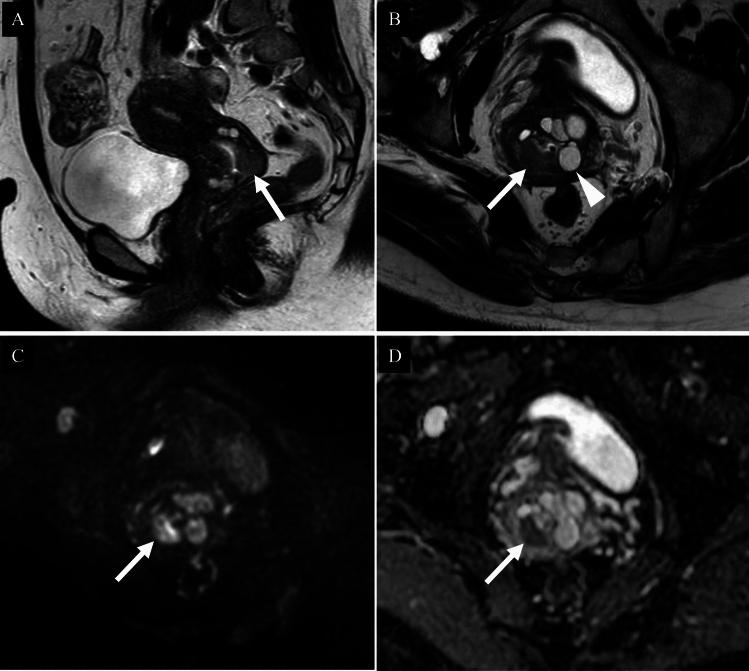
Clear cell carcinoma of the uterine cervix in a woman in her forties. A Sagittal T2-weighted image; B Axial T2-weighted image; C Axial diffusion-weighted image; D Axial apparent diffusion coefficient map. A T2-hyperintense mass is found to be localized to the posterior wall of the uterine cervix (A, B: arrows); the mass also shows restricted diffusion (C, D: arrows), with an ADC of 0.989 × 10⁻^3^ mm^2^/s. The surrounding cervical stroma is preserved. Multiple adjacent cysts are noted (B: arrowhead); however, these findings are consistent with those of Nabothian cysts rather than tumor-associated cysts. Differentiation from squamous cell carcinoma based on imaging findings alone is difficult. The tumor was pathologically diagnosed as an FIGO 2018 stage IB1 clear cell type HPV-independent adenocarcinoma
